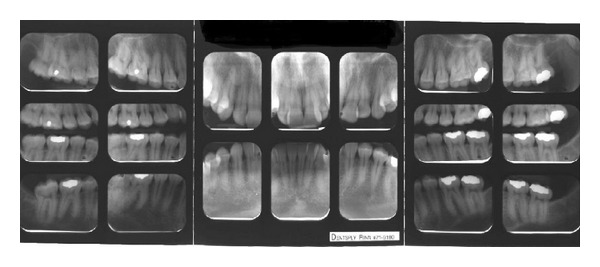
Initial full mouth radiographs.
